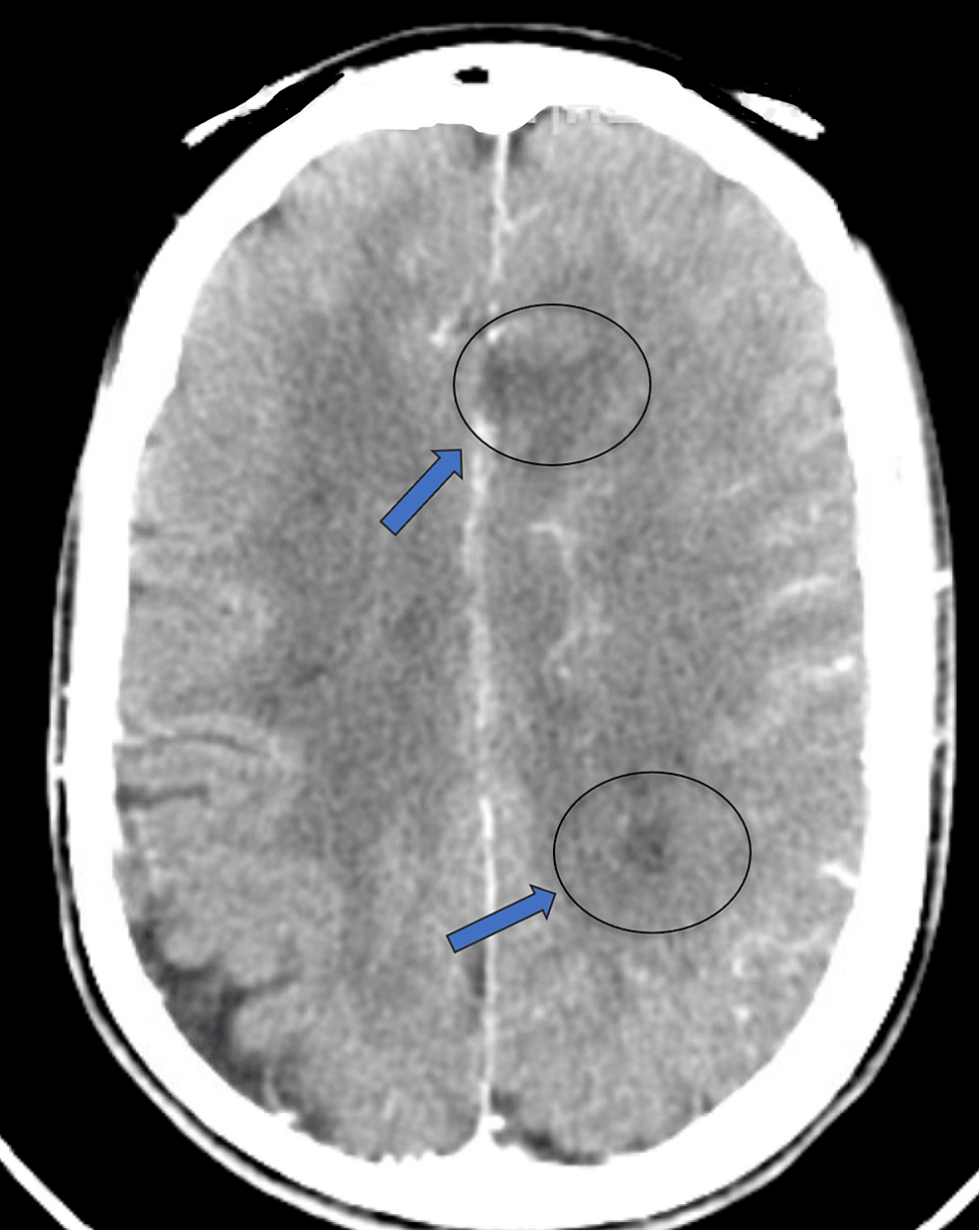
A follow-up CT scan conducted 48 hours post-mechanical thrombectomy indicating the presence of additional infarctions (blue arrows).
